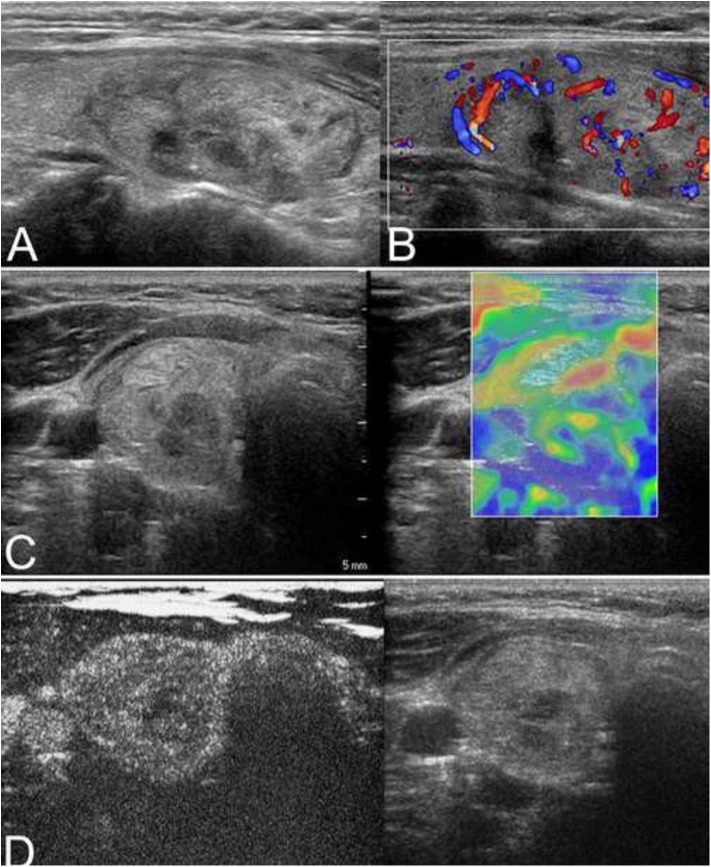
A nodular goiter with adenomatous hyperplasia in a 59-year-old woman is shown. (A) Conventional ultrasonography (CUS) showed that the solid hyperechoic nodule in the right lobe was heterogenous in echotexture and had regular margins, indicative of low suspicion. (B) Color Doppler showed intranodular and peripheral vascularity. (C) The elastography score was 2, indicating a soft stiffness. (D) Contrast-enhanced ultrasonography (CEUS) revealed ring-enhancement.
